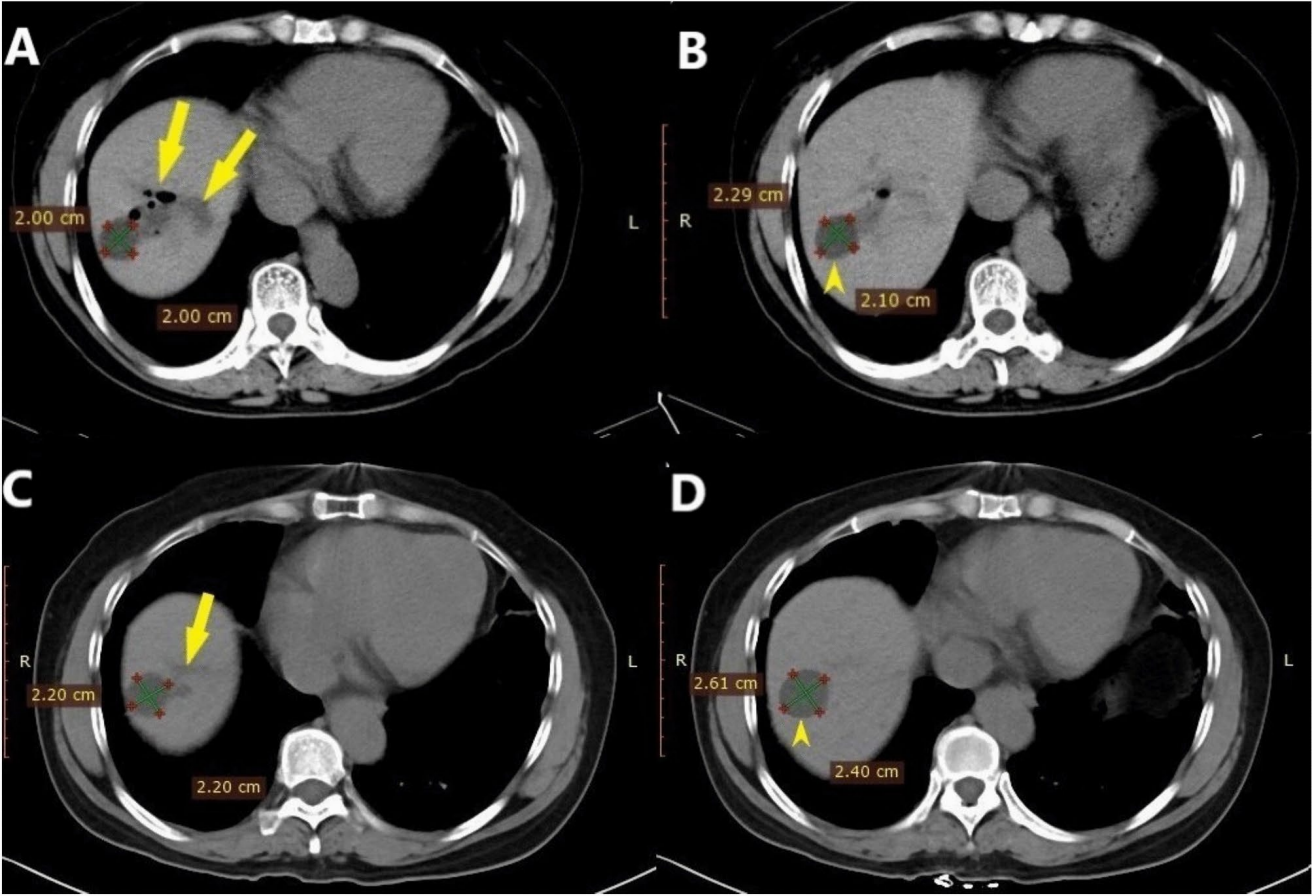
The patient did not complain of pain during injecting ethanol. The patient was discharged after 3 days without complications. Comparison images of immediate and 6-month postprocedural follow-ups. (A, B) In December 2019, CT performed immediately after the procedure revealed that the treated large cyst was significantly reduced in size with a small amount of gas remaining (yellow arrow) on consecutive scanning levels. The size of the untreated small cyst was 2.3 cm × 2.1 cm (yellow arrowhead). (C, D) In June 2020, CT performed after 6 months demonstrated that the treated large cyst had completely regressed with only the collapsed cyst wall remaining, and the untreated small cyst (2.6 cm × 2.4 cm) was slightly enlarged.
